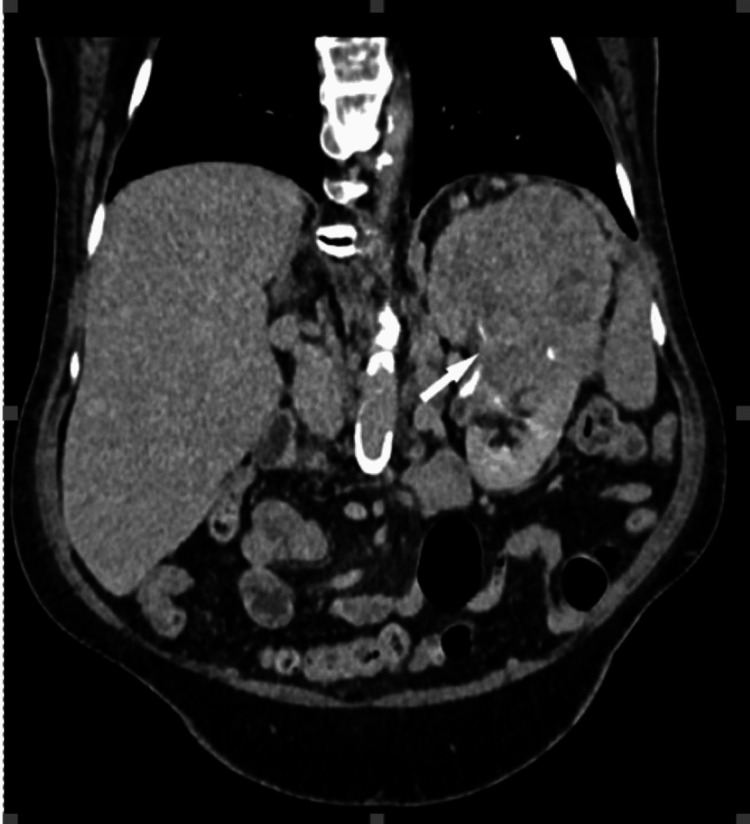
Coronal oblique urographic contrast-enhanced CT (CECT) phase presents a striking visual representation of a substantial mass originating from the upper two-thirds of the left kidney. This voluminous lesion exhibits characteristics indicative of necrosis, further emphasizing its significant size and advanced pathology. Notably, the mass is depicted invading the renal sinus. This figure is the original work of the authors. Patient consent for the use of the image was obtained, as mentioned in the patient consent form (point no. 12).
